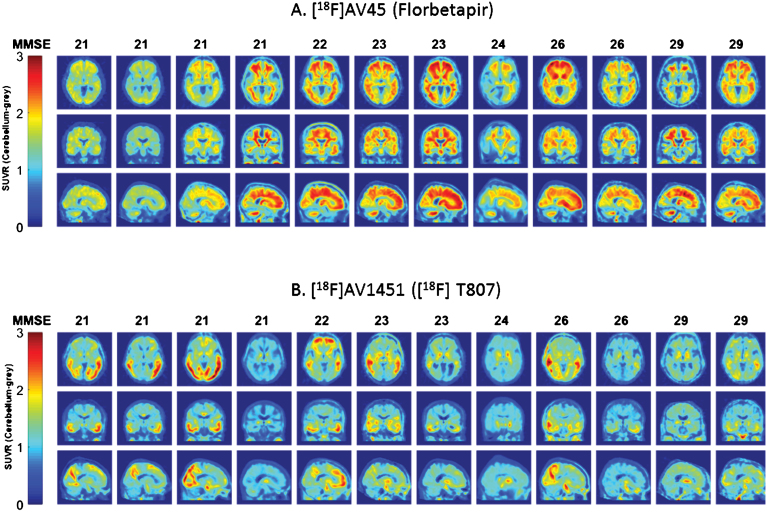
Topographical plots of PET SUVr levels for the 12 participants who underwent both Aβ and tau imaging. A and B demonstrate, respectively, [^18^F]AV45 (Aβ) and [^18^F]AV1451 (tau) topographical distribution of SUVr signal across the 12 participants (each column represents a participant; participants have been ordered according to baseline MMSE scores).
